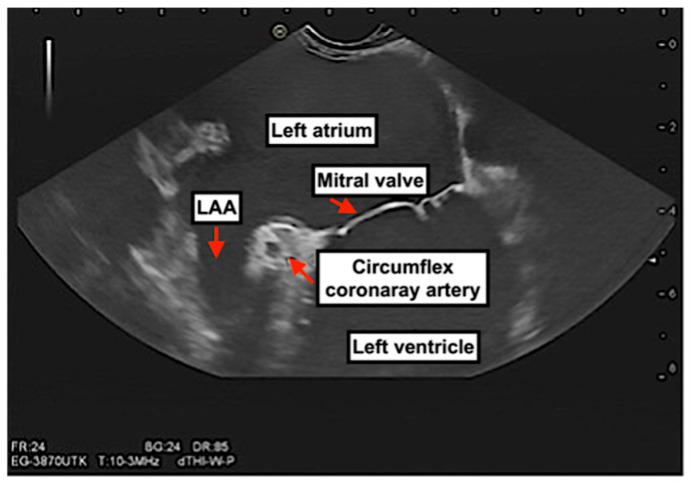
Endoscopic ultrasound demonstrated the morphology of the mitral valve, circumflex artery, and left atrial appendage.
